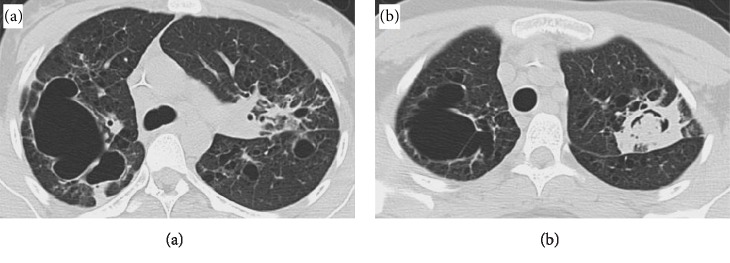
Computed tomography of multiple bilateral bullae and lung cavitations. (a) Largest bullae measuring up to 8 cm in the right upper lobe and filled with air. (b) Suspected mycetoma within the left upper lobe lung cavitation.
